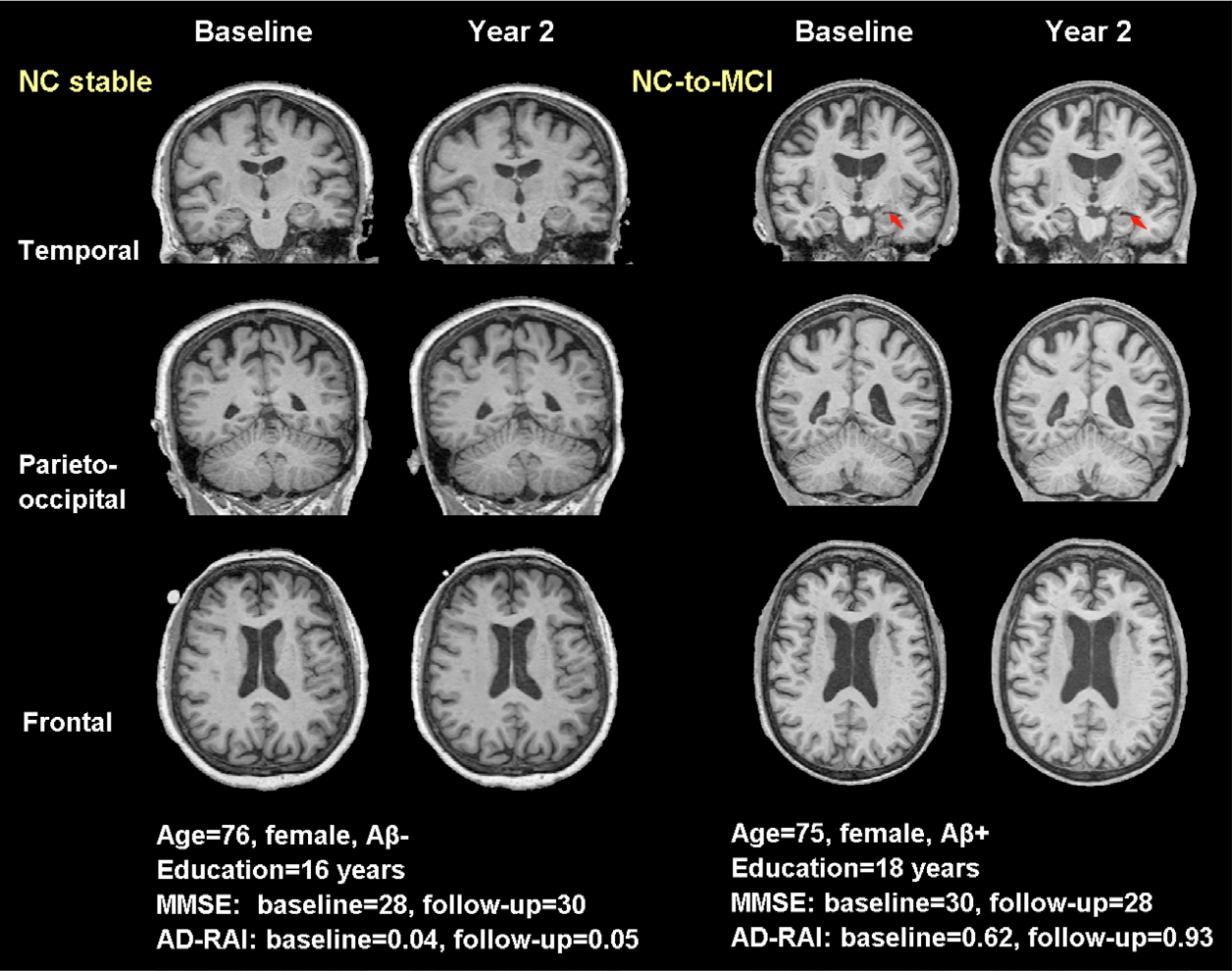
Typical real cases of NC stable and NC-to-MCI subjects. T1-weighted (T1W) images at baseline and two years were shown for the two typical cases in temporal, parieto-occipital and frontal view. Red arrows pointed to the region with significant atrophy by comparing the T1W images of the same subject over two years. The typical case of NC stable did not present atrophy while the case of NC-to-MCI showed increased width of left choroid fissure and temporal horn (temporal view). Aβ-: CSF-based Aβ_42_ >192 pg/ml at baseline and 2 years; Aβ+: CSF-based Aβ_42_ <192 pg/ml at baseline and 2 years; AD-RAI: AD resemblance atrophy index.
